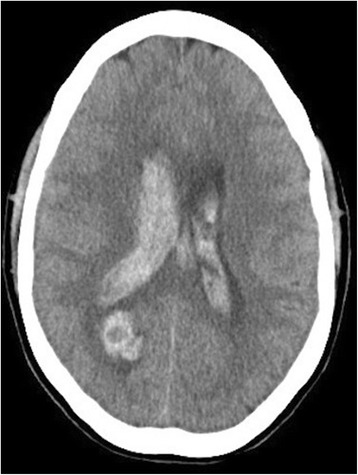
CT image showing a subcortical hematoma in the right occipital lobe, along with bilateral intraventricular hematomas
